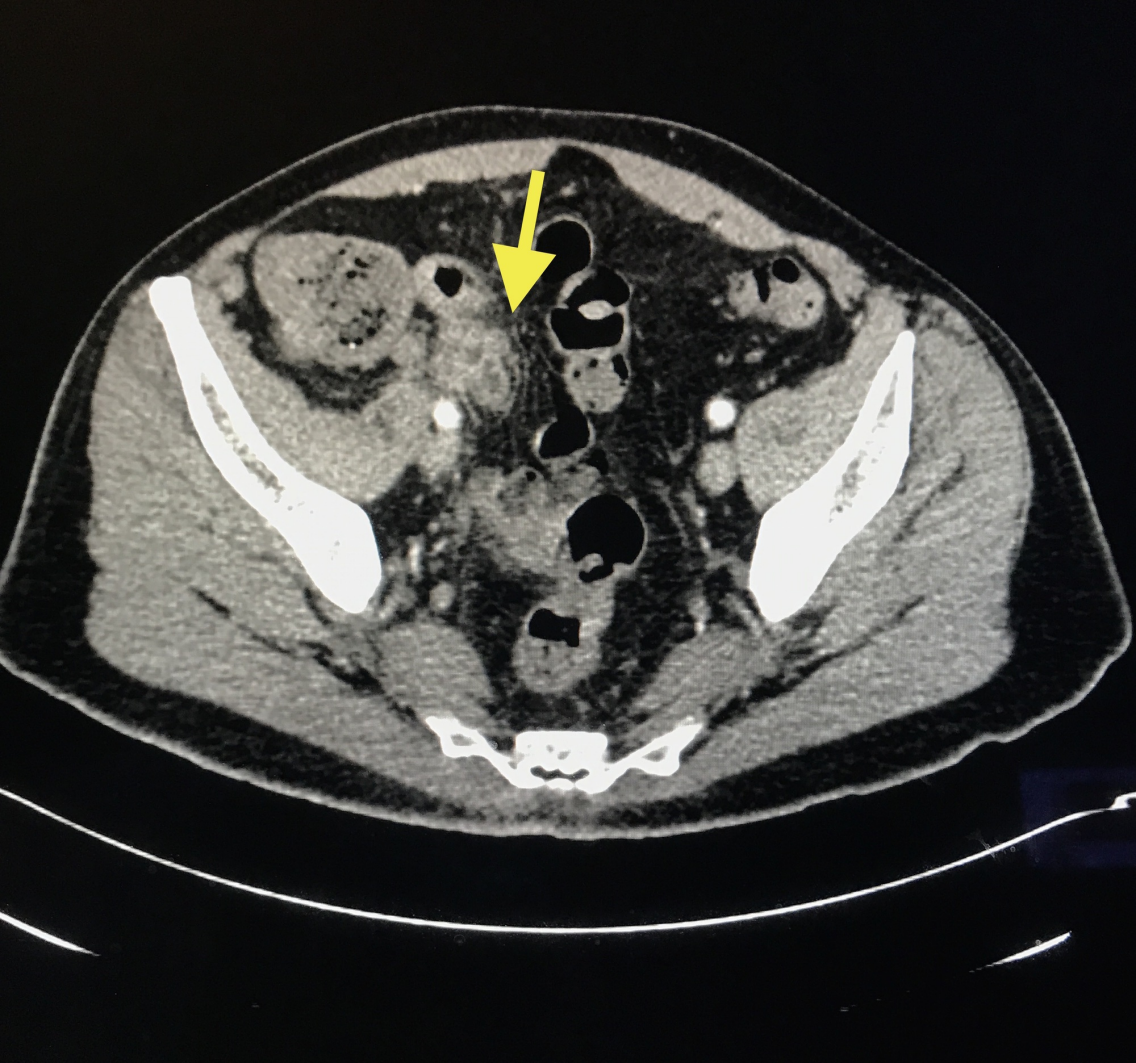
CT scan of the abdomen/pelvis showing mass measuring 3 cm at the tip of the appendix (yellow arrow), with the absence of collection Findings suspicious for post-inflammatory changes within the area; however, malignancy cannot be excluded. CT: computed tomography
